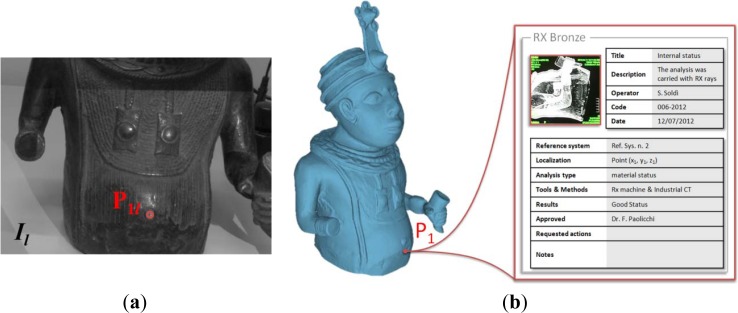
(a) 2D selection of a reference point on the left stereo image. (b) Radiographic analyses associated to the virtual model by using the correspondent 3D point.
